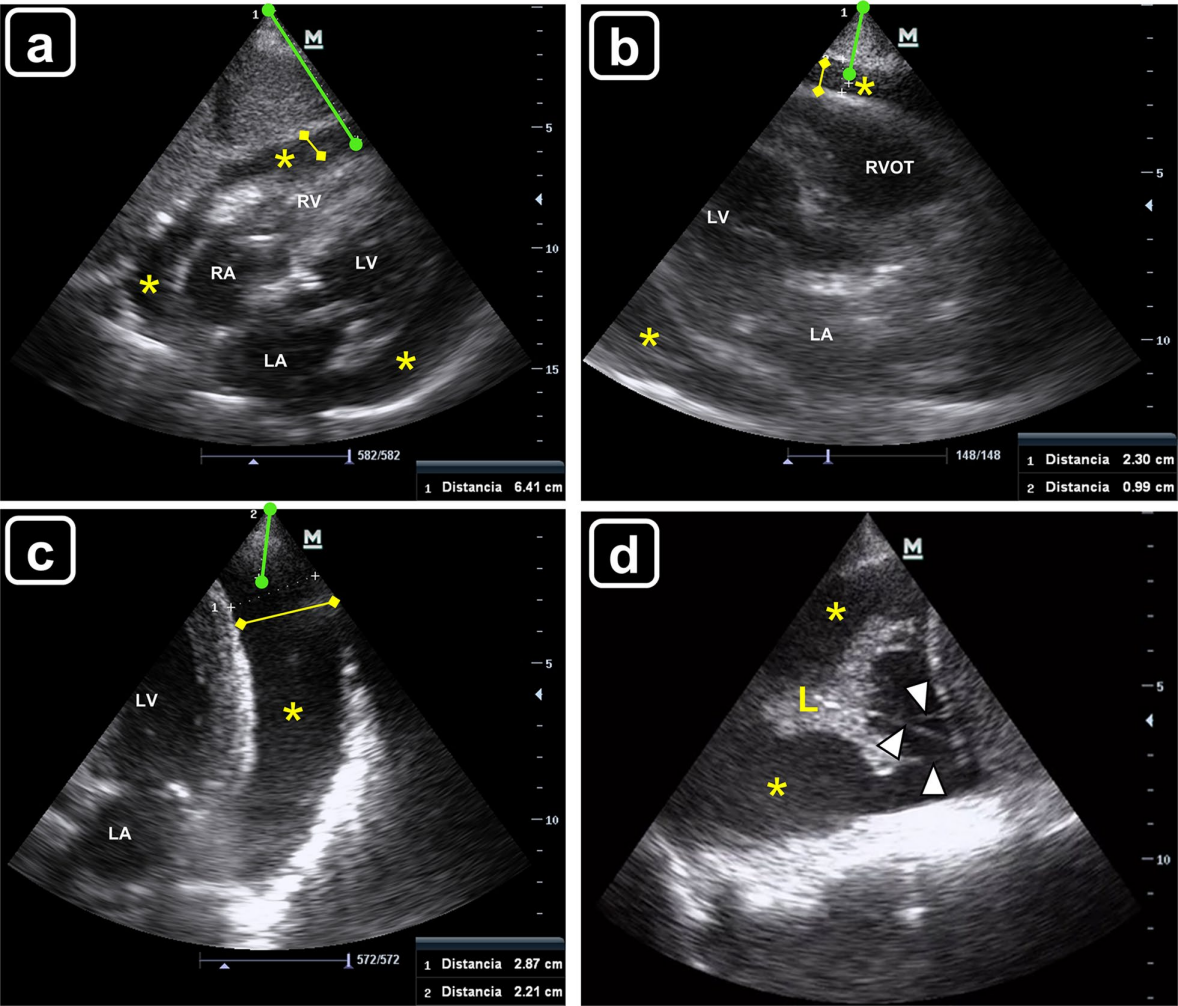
Point-of-care echocardiogram showing the circumferential pericardial effusion (asterisks), the pericardial fluid thickness (yellow line) and the distance to reach the pericardial cavity from the skin (green line); a subcostal four chamber view, b parasternal long axis view, c apical four chamber view, *RA* right atrium; *RV* right ventricle; *LA* left atrium; *LV* left ventricle; *RVOT* right ventricular outflow tract. d Left-sided pleural effusion (asterisks) with fibrin strands (arrowheads); *L* lung consolidation
